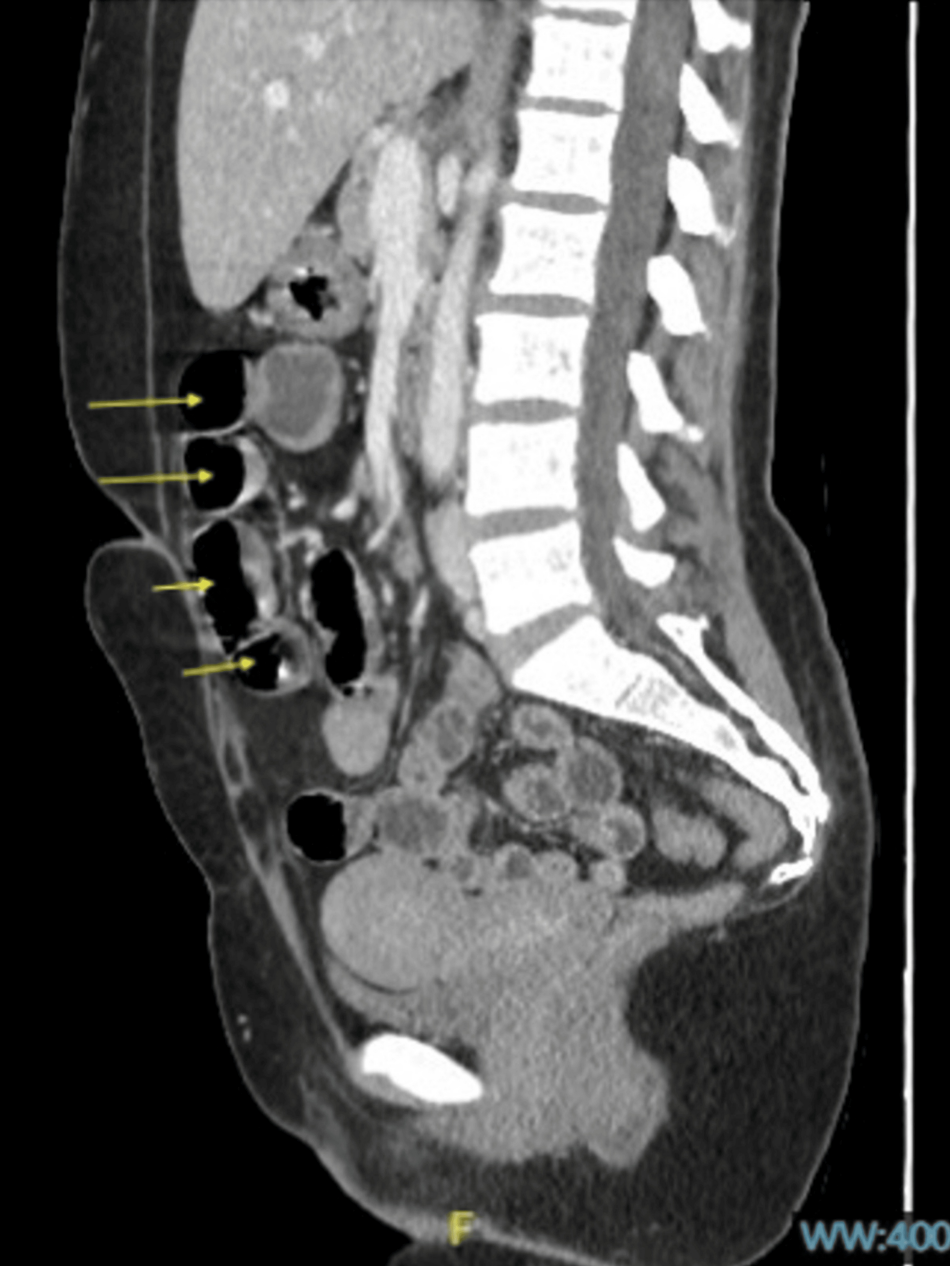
Abdominal CT scan demonstrating colonic pseudo-obstruction denoted by yellow arrows.
